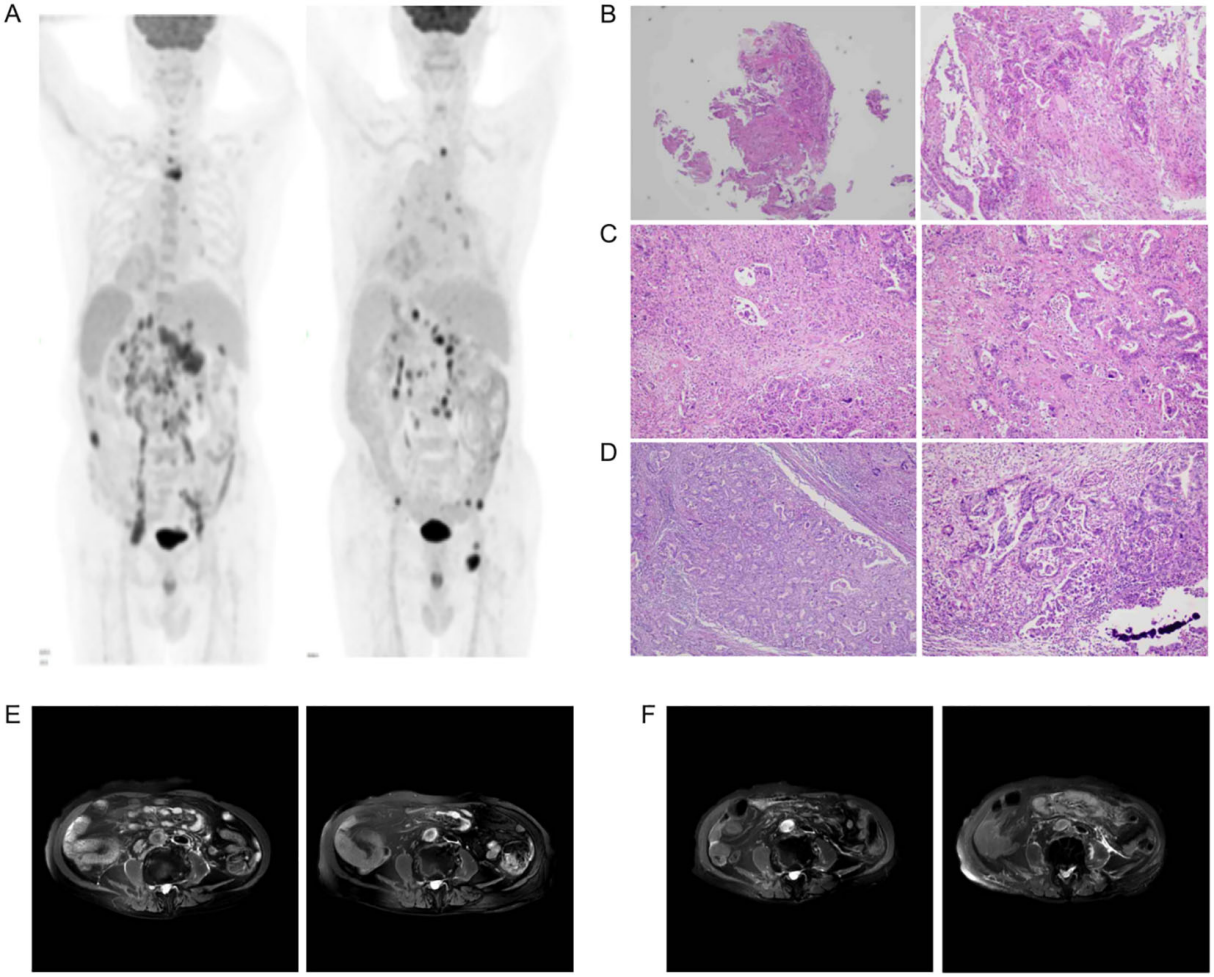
PET-CT [*2023-11 left vs 2024-04 right*, (A)]. Endoscopic biopsy from gastric antrum and duodenal bulb under endoscope [2023-11, (B), *left×100, right×400 right*]. Hepatic lesion resection [2023-11, (C), *left×100, right×400 right*]. Left inguinal lymph node [2024-04, (D), *left×100, right×400 right*]. Abdominal MRI T2 [*2023-10 left vs 2024-01 right*, (E)]. Abdominal MRI T2 [*2024-06 left vs 2024-12 right*, (F)].
